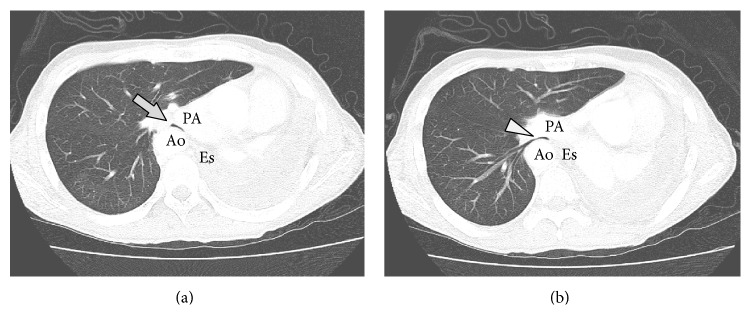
Computed tomography taken after the development of postpneumonectomy syndrome. Extremely narrowed bronchus intermedius ((a) arrow) and right lower bronchus ((b) arrowhead) are shown, compressed between the right pulmonary artery and the right descending aorta. Ao, right descending aorta; PA, right pulmonary artery; Es, esophagus.
